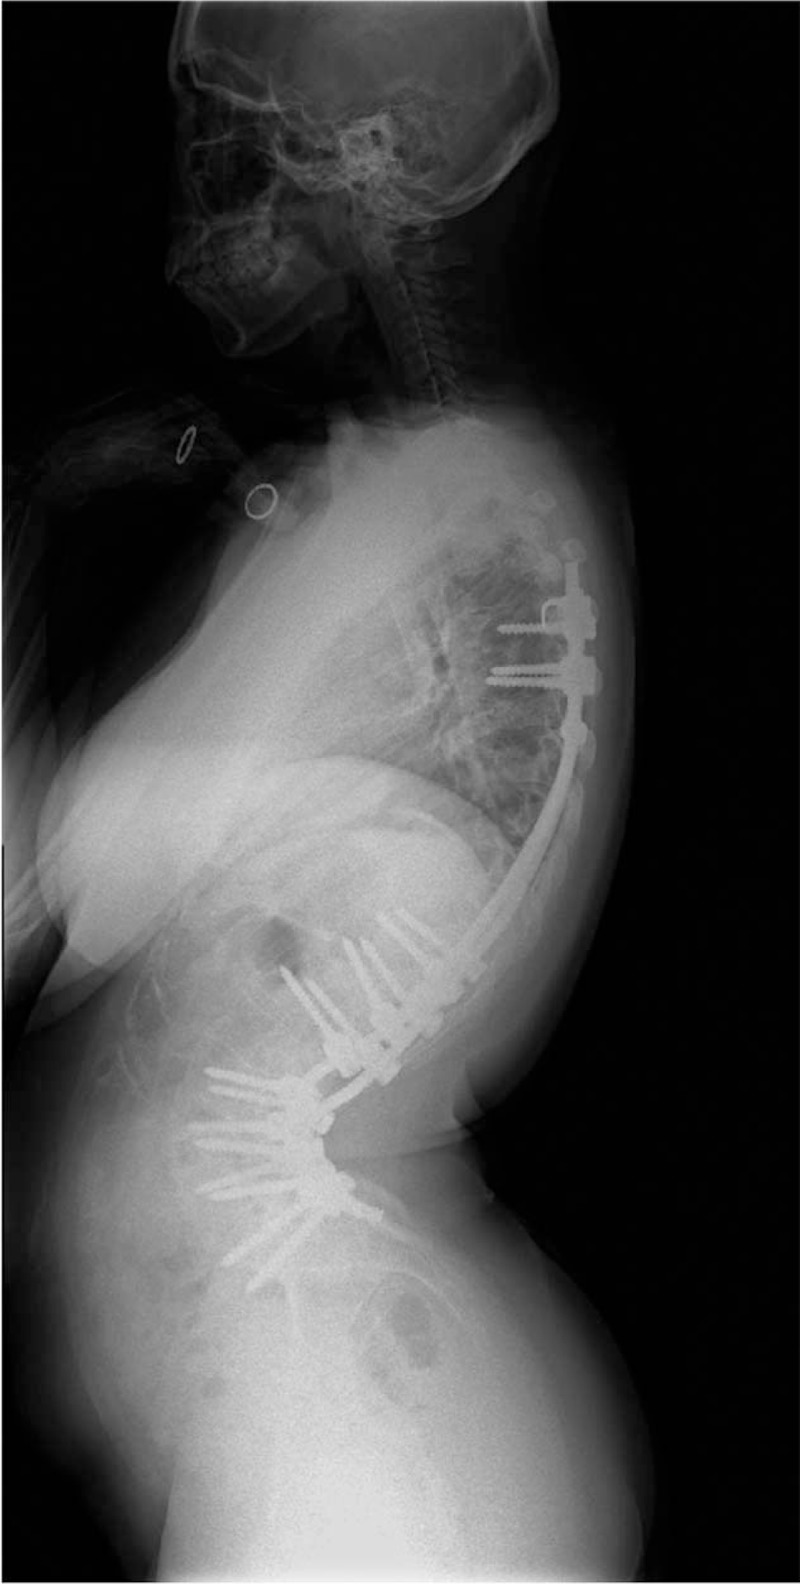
Postoperative lateral radiograph showing the hyperlordosis curve corrected to 93° and sagittal balance corrected to −50 mm.
